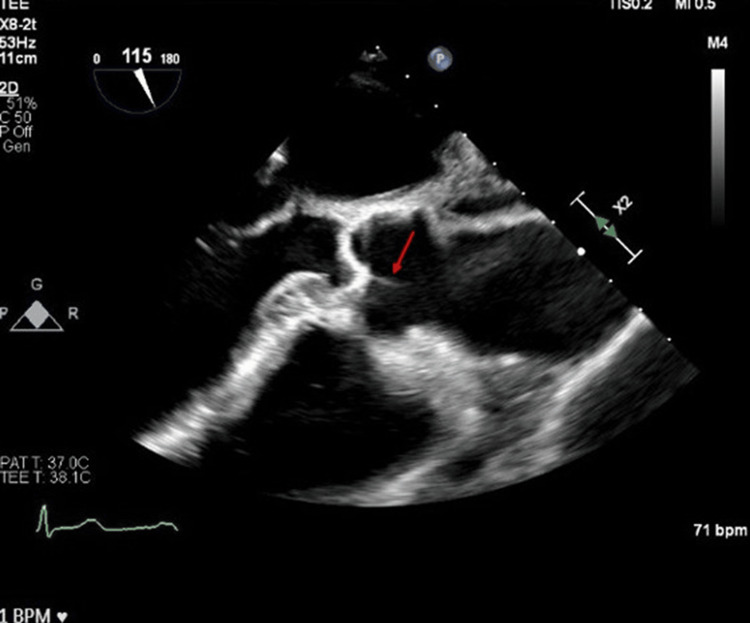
Transesophageal echocardiograph showed normal left ventricular (LV) function, trace aortic regurgitation with Lambl’s excrescence noted (red arrow), and mild mitral valve regurgitation
